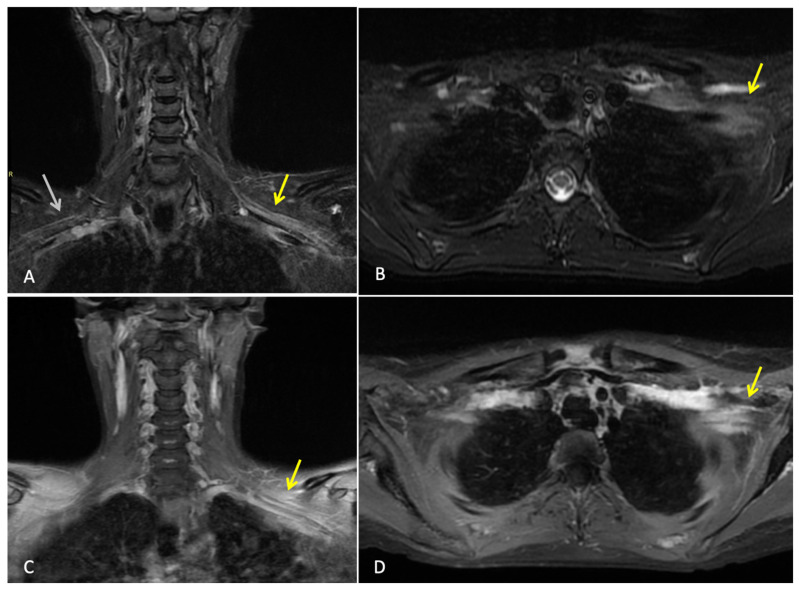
MRI brachial plexus: This is a figure. Images (A,B) are coronal and axial T2 STIR images that demonstrate thickening and increased T2 signal of the brachial plexus on the left (yellow arrows). Normal size and signal of the brachial plexus on the right for comparison (white arrow). Images (C,D) are coronal and axial T1 fat-saturated post-contrast images, which demonstrate abnormal hyperenhancement of the thickened brachial plexus on the left side (yellow arrows). Findings are consistent with left brachial plexitis.
